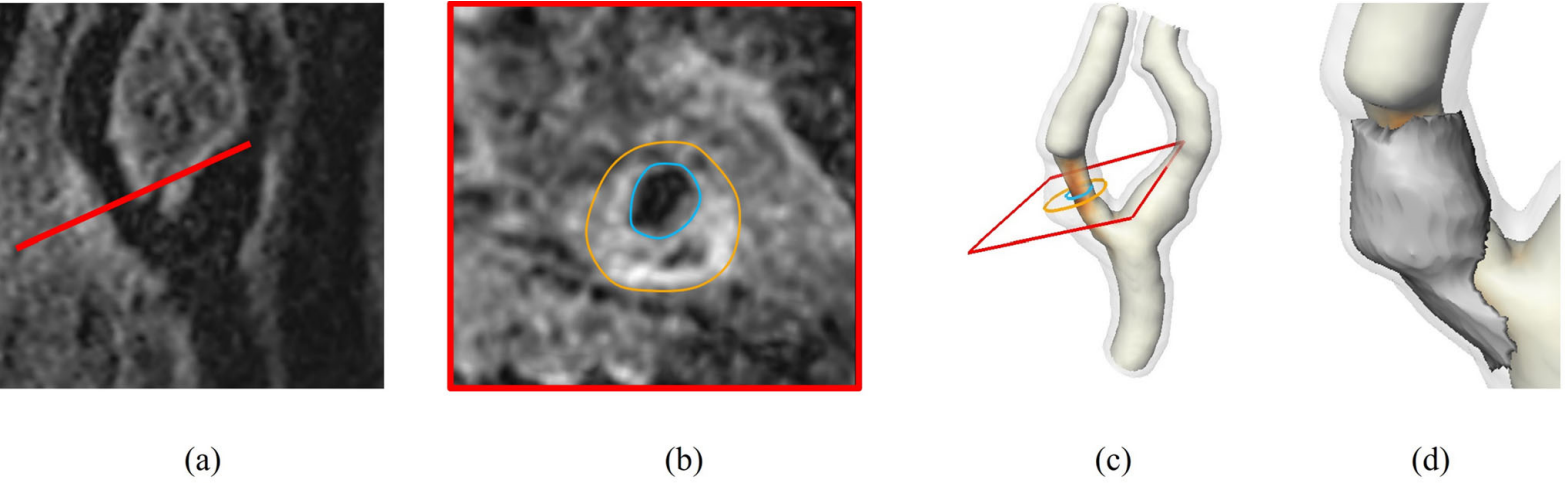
a View of an atherosclerotic carotid artery in T1-weighted 3D BB-MRI with cross-section MPR. b Segmented 2D cross section of the carotid artery which can be used for the computation of wall area and maximum wall thickness. c 3D segmentation of the carotid wall with the VWT color-coded on the lumen surface. d Lesion extracted from the 3D segmentation which can be used for the quantification of lesion volume, surface, and compactness
